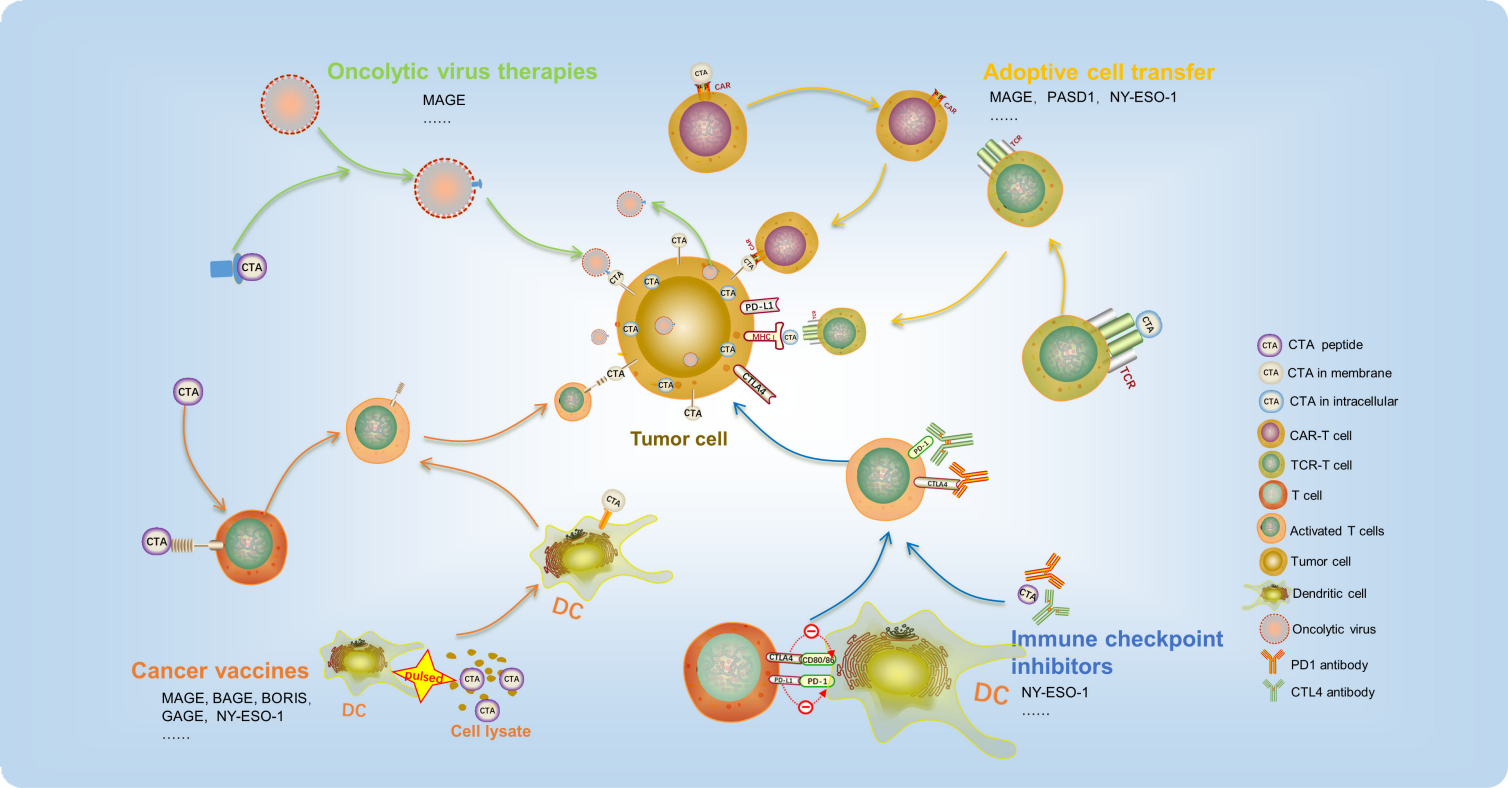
CTA-based immunotherapy has recently been used in cancer treatment and achieved promising outcomes. It mainly includes oncolytic virus therapies, cancer vaccines, adoptive cell transfer, and immune checkpoint inhibitors.
